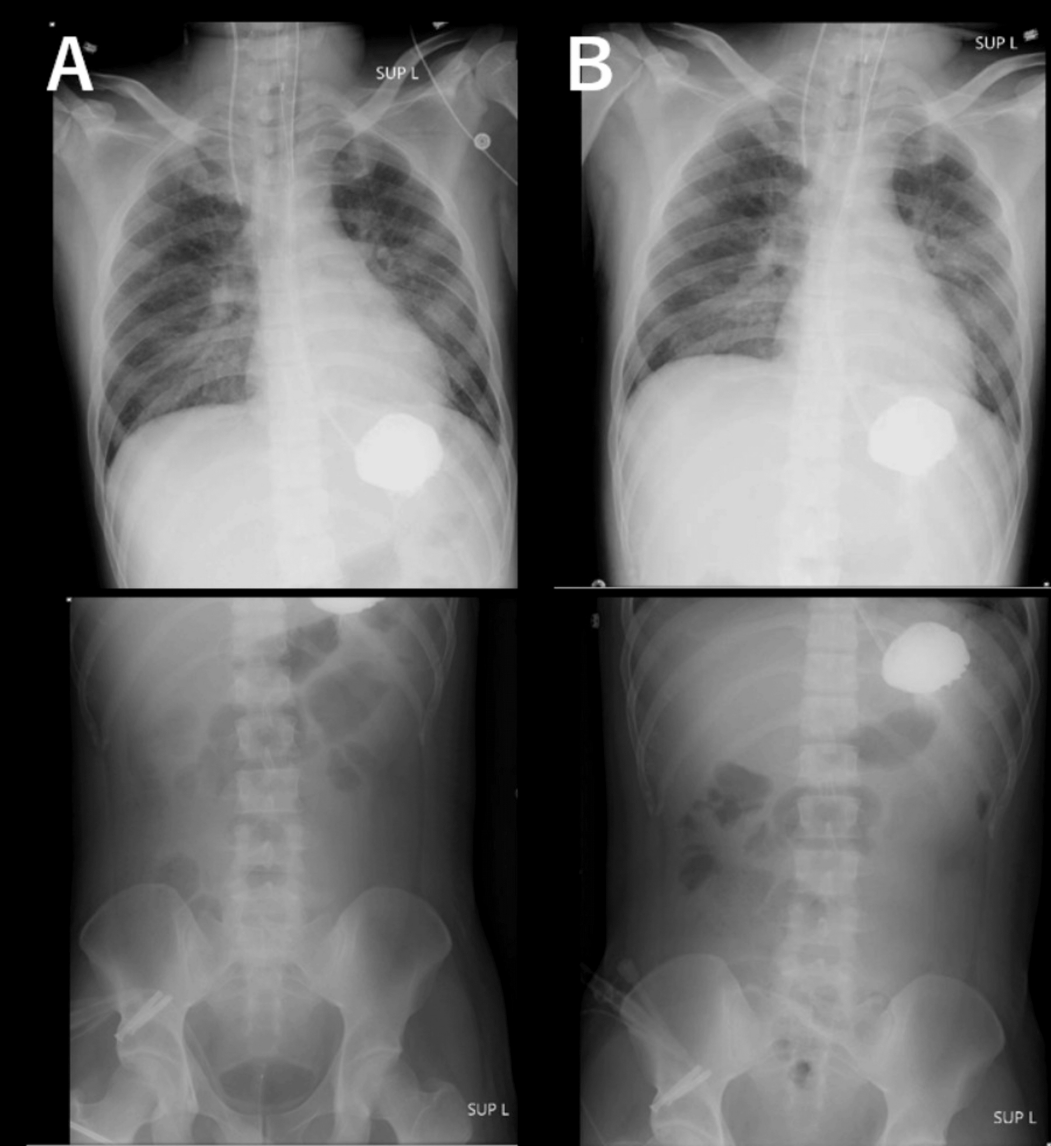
Post-procedure chest and abdominal X-rays A: X-rays taken immediately after gastric lavage and before Gastrografin administration. B: X-rays taken 12 h after Gastrografin administration.
